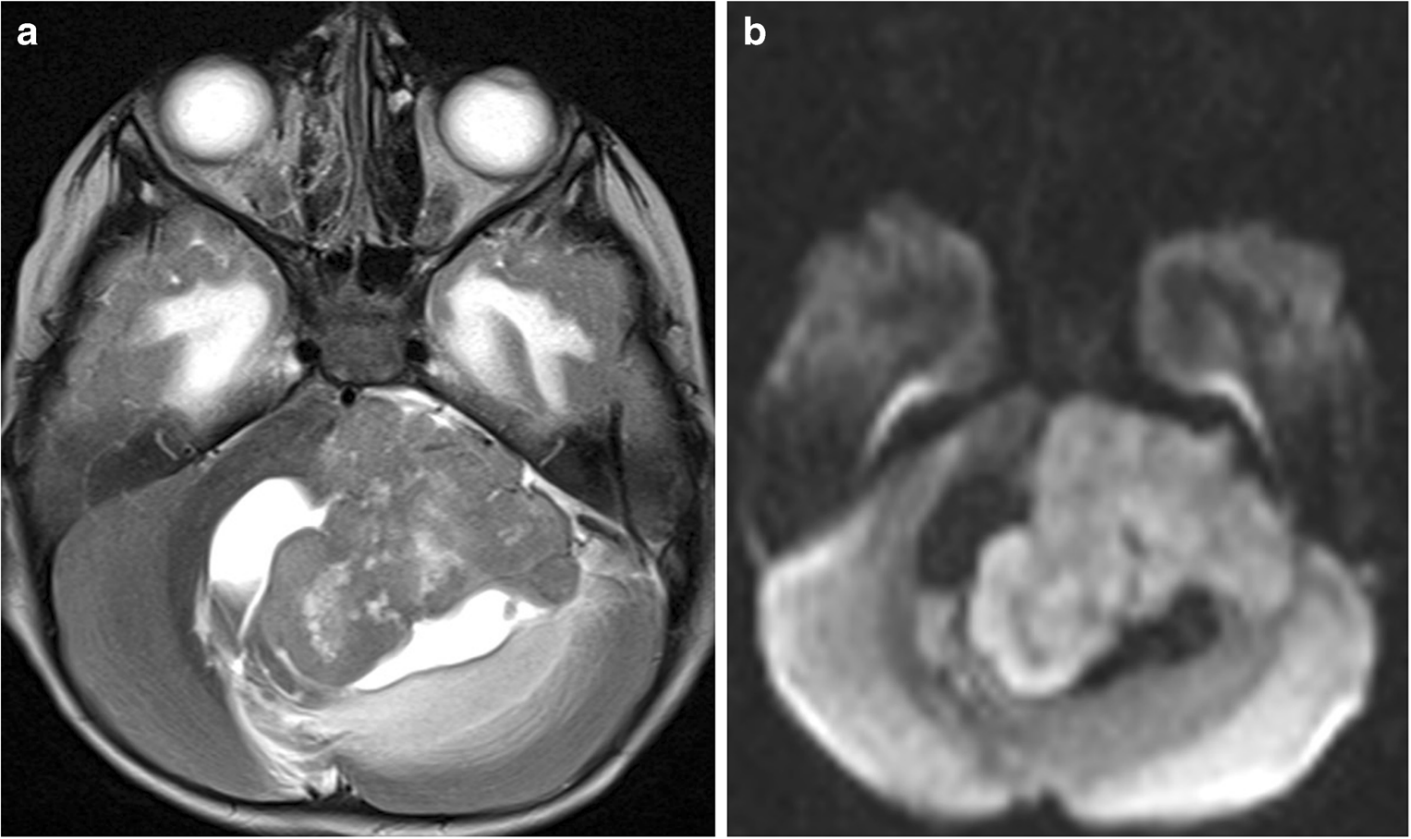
A 5-year-old patient with cerebellar medulloblastoma: a–b Axial T2-weighted and diffusion-weighted image shows a large hypointense mass showing restriction in the left cerebellar hemisphere causing severe brainstem compression
